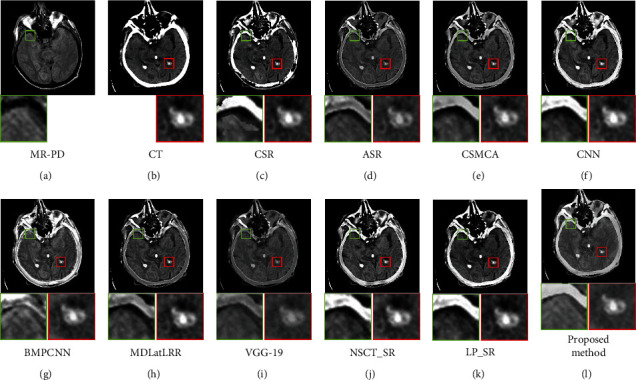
Comparison of the fusion results in the first set images. (a) MR-PD. (b) CT. (c) CSR. (d) ASR. (e) CSMCA. (f) CNN. (g) BMPCNN. (h) MDLatLRR. (i) VGG-19. (j) NSCT_SR. (k) LP_SR. (l) Proposed method.
